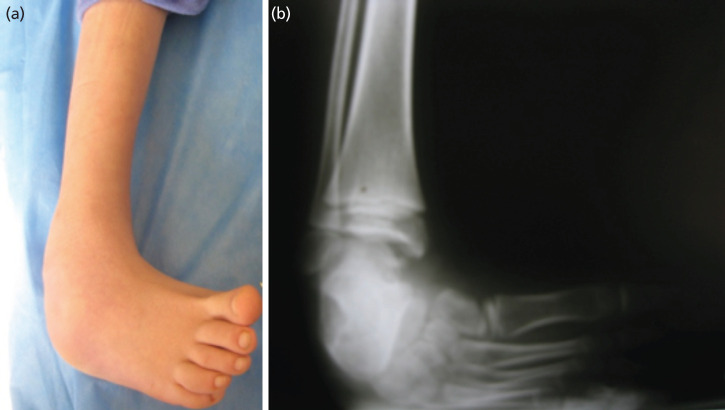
(a, b) Pre-operative radiograph and photograph of a patient with clubfoot.
